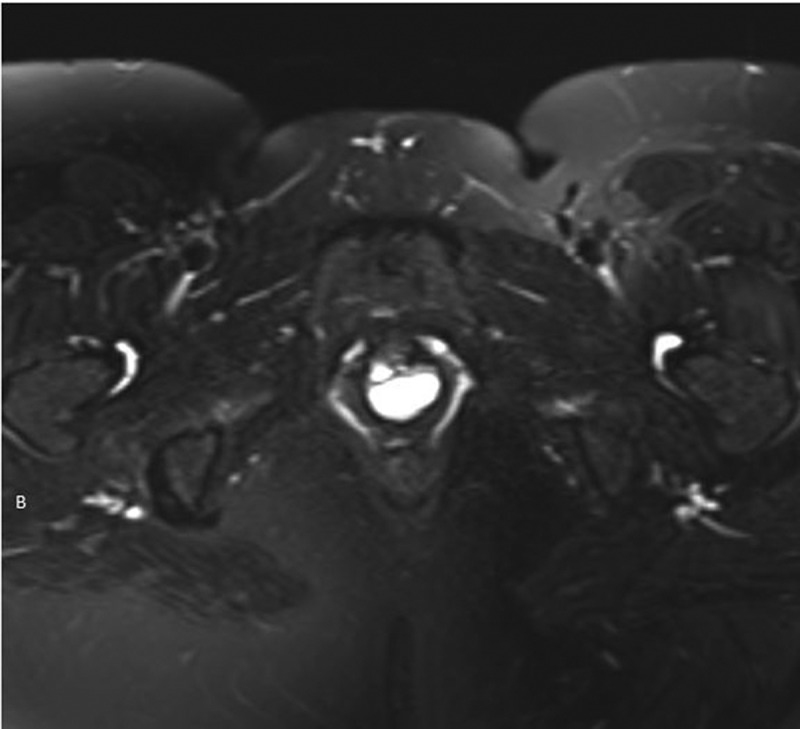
Axial T2-weighted MRI image of a 1.8-cm simple UD.
